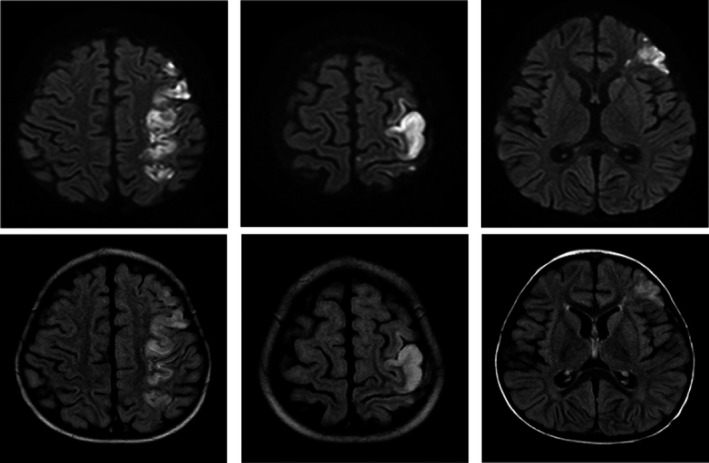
Magnetic resonance imaging of abnormal restricted diffusion/infarct within the left middle cerebral artery and watershed distribution (day 4 of hospitalization). DWI (top) and T2 FLAIR (bottom)
